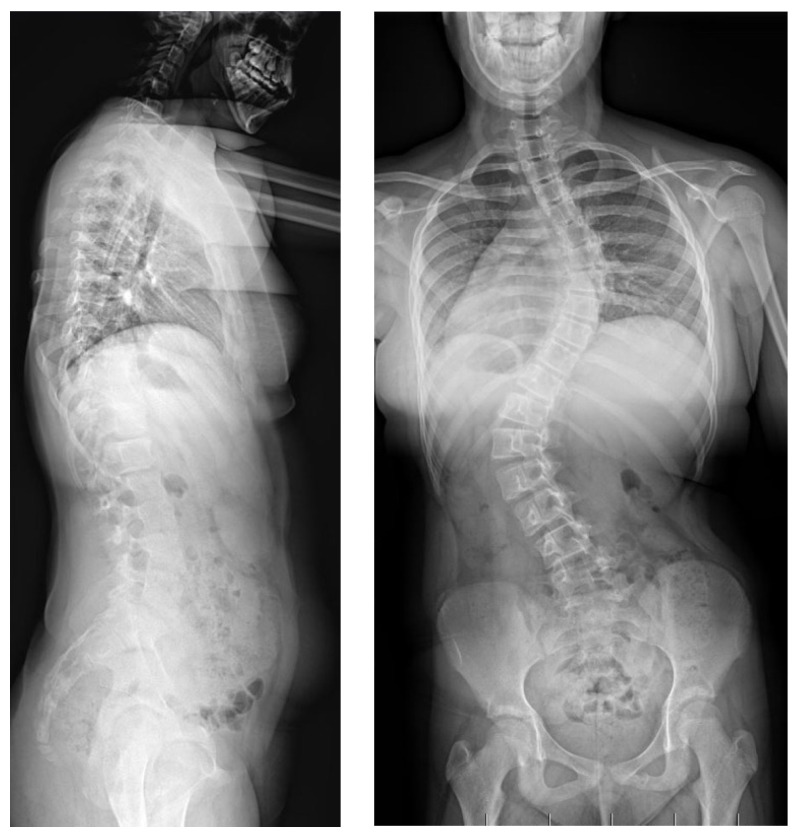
Preoperative images in a standing position: (from (left) to (right)) LAT and AP X-rays at 9 years old (76 degrees for the main curve).
